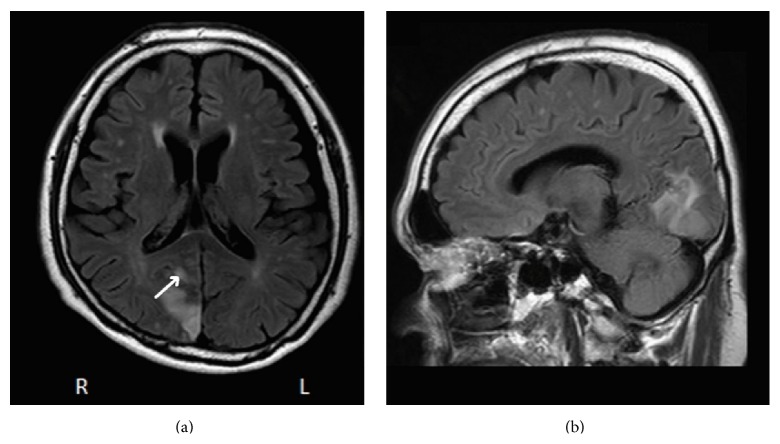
FLAIR MRI scan of the case on (a) axial and (b) sagittal views. High-signal intensity can be seen in the right posteromedial region that extended into the anterior bank of the parietooccipital sulcus (arrow). FLAIR: fluid attenuated inversion recovery; MRI: magnetic resonance imaging.
